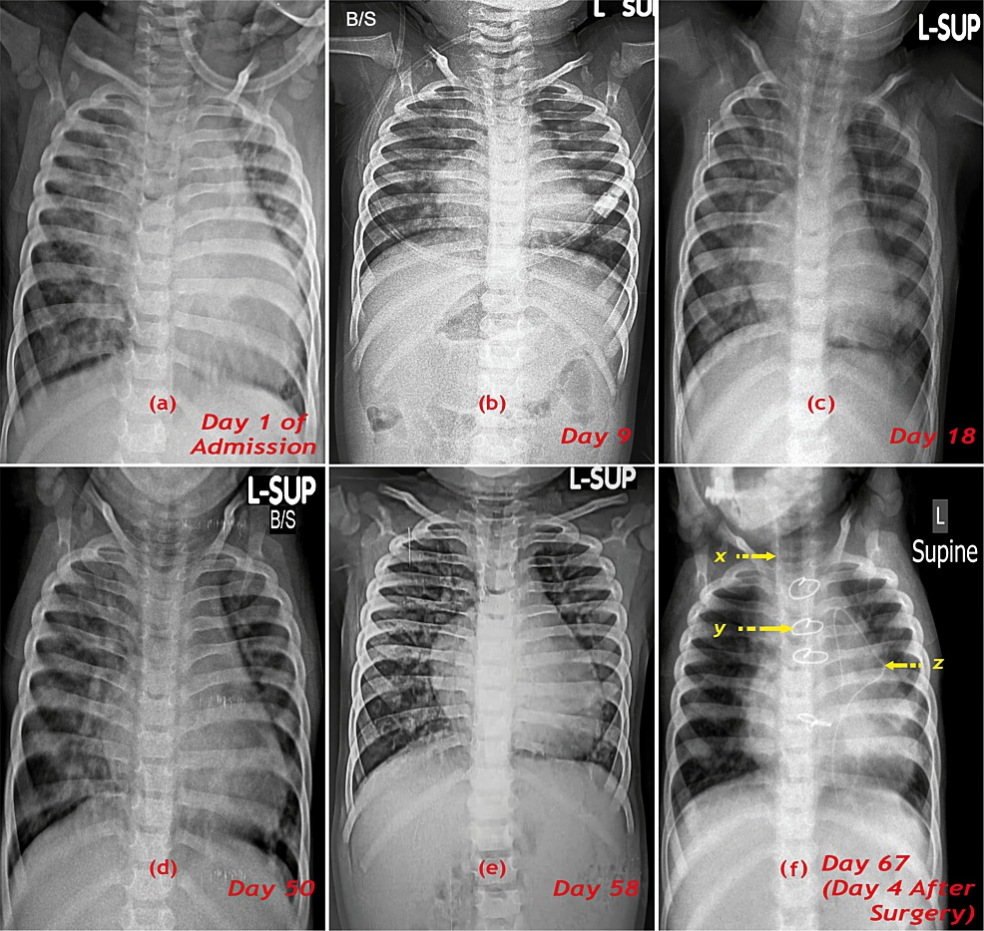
Chest X-rays at various stages from the day of admission till post-cardiac surgery depicting the resolution of bronchopneumonia. x: central venous pressure line; y: sternal wires; z: pacing wire Image credits: Dr. Vishal V. Bhende
